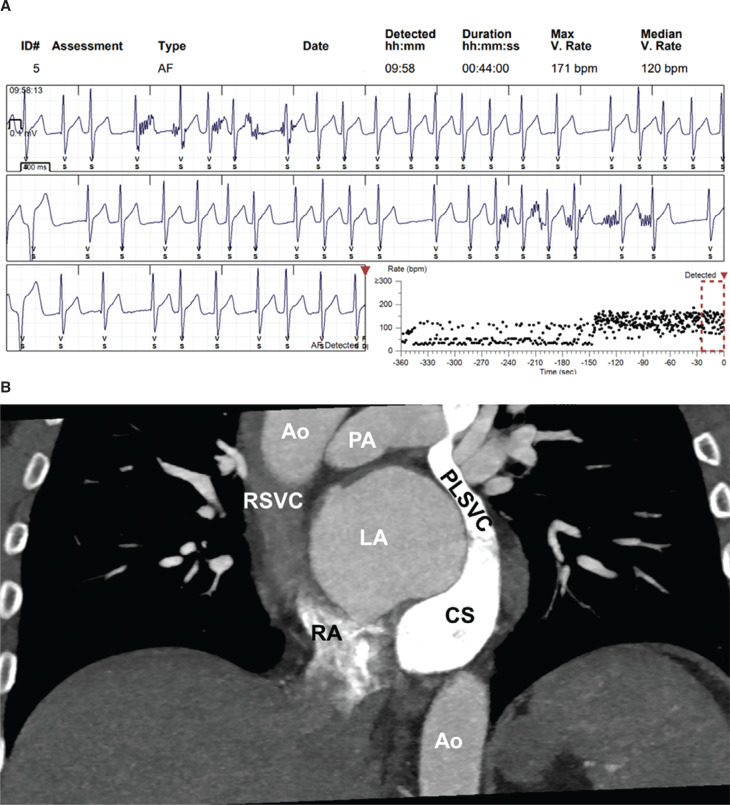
A: Implantable cardiac monitor recording of an episode of atrial fibrillation. B: Cardiac computed tomography angiography oblique view showing the patient’s persistent left superior vena cava and right superior vena cava. *Abbreviations:* AF, atrial fibrillation; Ao, aorta; CS, coronary sinus; LA, left atrium; PA, pumonary artery; PLSVC, persistent left superior vena cava; RA, right atrium; RSVC, right superior vena cava.
